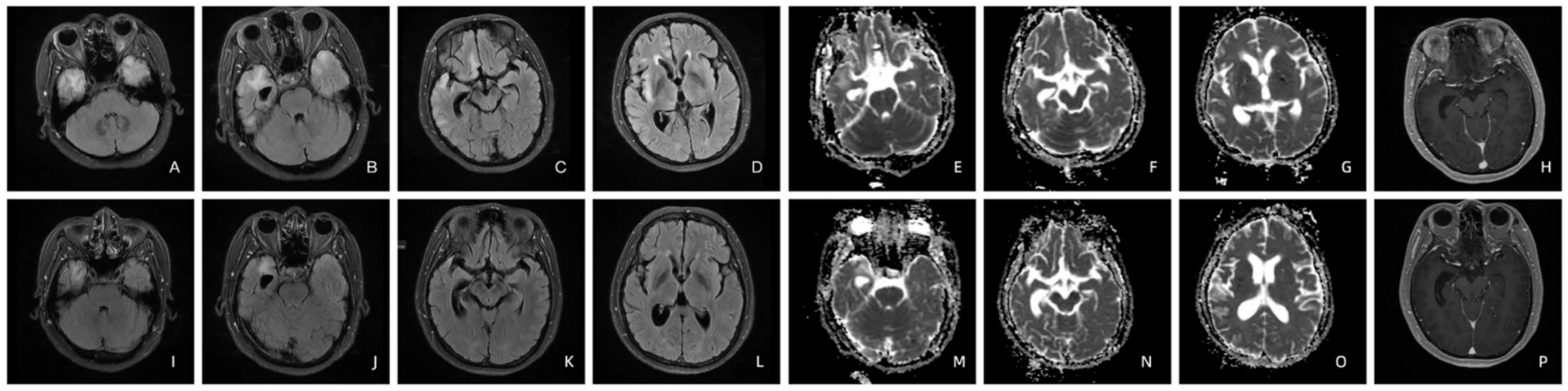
T2-FLAIR imaging of patient 6 before (A–D) and four months after treatment (I–L). The ADC (E–G) and contrast-enhanced T1-weighted imaging (H) of patient 6 before and four months after treatment (M–P).
